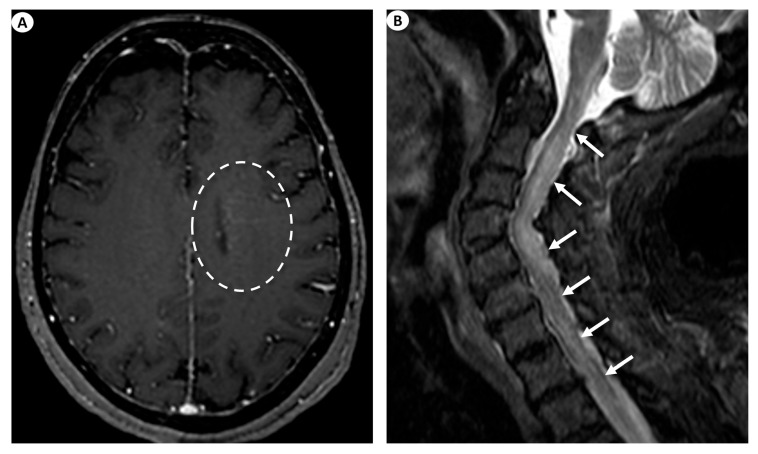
(A) Axial T1-weighted contrast-enhanced images following intravenous gadolinium injection demonstrate subtle periventricular linear contrast enhancement on the left cerebral hemisphere extending outwards in a radial pattern in a patient with GFAP astrocytopathy (dashed oval). (B) Sagittal fat-saturated T2-weighted images display abnormal signal hyperintensity extending craniocaudally from the cervicomedullary junction throughout the whole cervical spinal cord to the thoracic spinal cord (more than three vertebral levels affected), findings indicative of longitudinally extensive myelitis (arrows) in a female patient with GFAP astrocytopathy.
